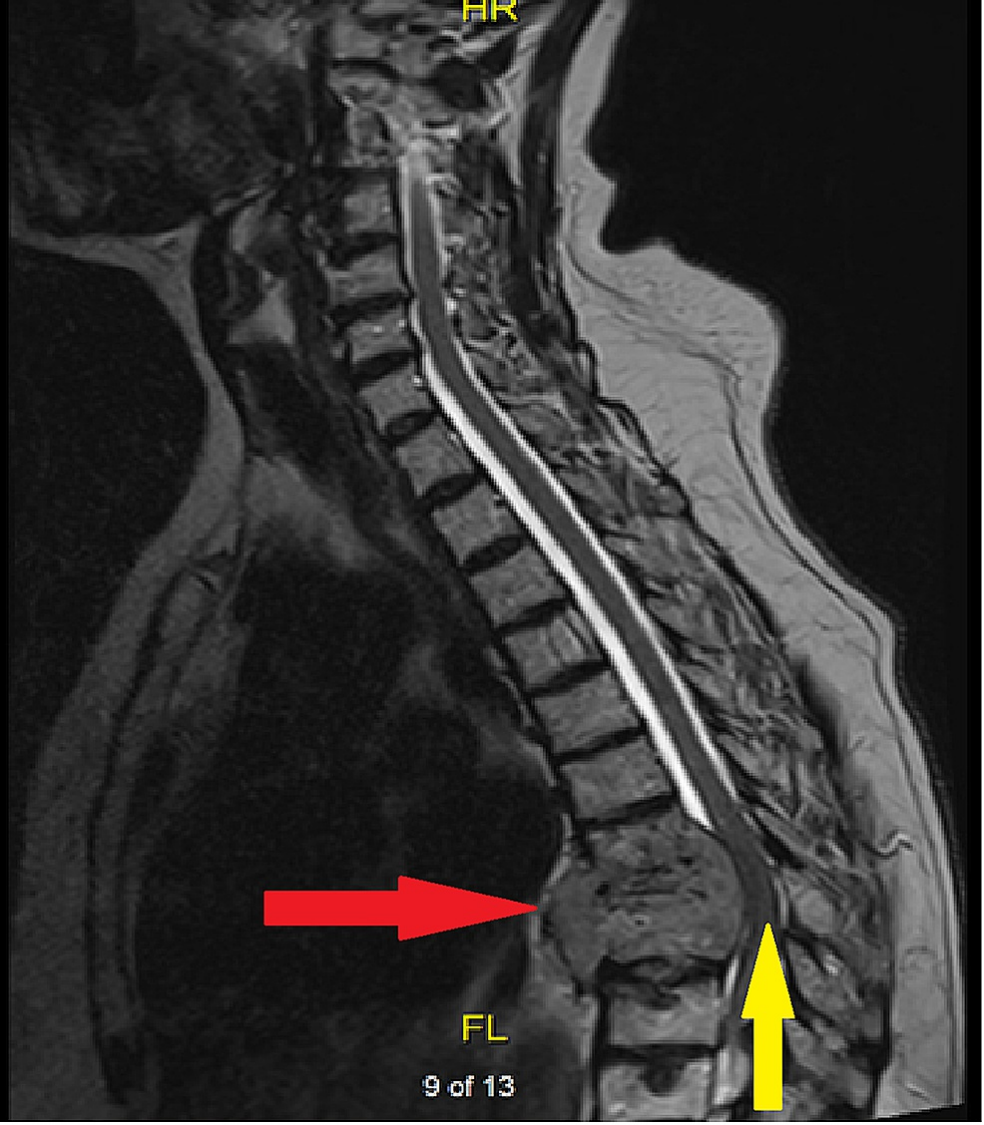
Plasmacytoma with spinal cord compression. Sagittal view of thoracic spine via T2 MRI. Red arrow indicating large plasmacytoma at the level of T7-T8. Yellow arrow indicating lack of contrast indicating cord compression as a result of the plasmacytoma
